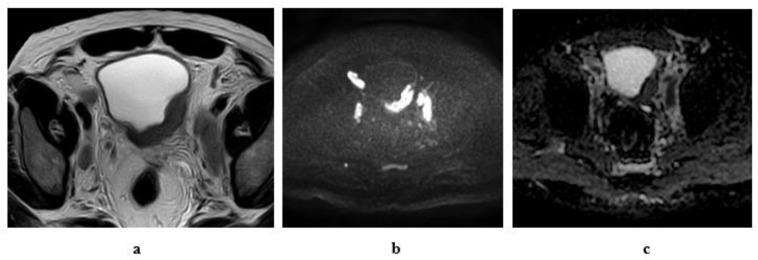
A 55-year-old male patient with an intravesical mass at the left posterolateral bladder wall (Micropapillary urothelial carcinoma, stage T3b N2): (a) high resolution T2 MRI: the soft tissue mass has an intermediate SI with infiltration of the perivesical fat, bilateral external and internal iliac lymphadenopathy. (b) Diffusion w MRI: b value 1400: restricted signals of the mass and bilateral LNs. (c) ADC of the mass = 0.8 × 10^−3^ mm^2^/s, of the lymph nodes = 0.9 × 10^−3^ mm^2^/s.
